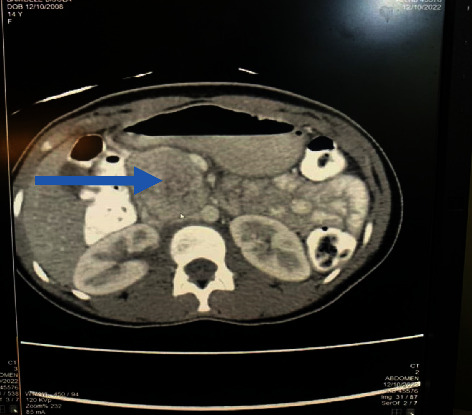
Contrast-enhanced axial computed tomography of the abdomen at the level of the kidneys showing an enhancing mass (arrow) just anterior and to the right of the aorta. It is compressing the inferior vena cava.
